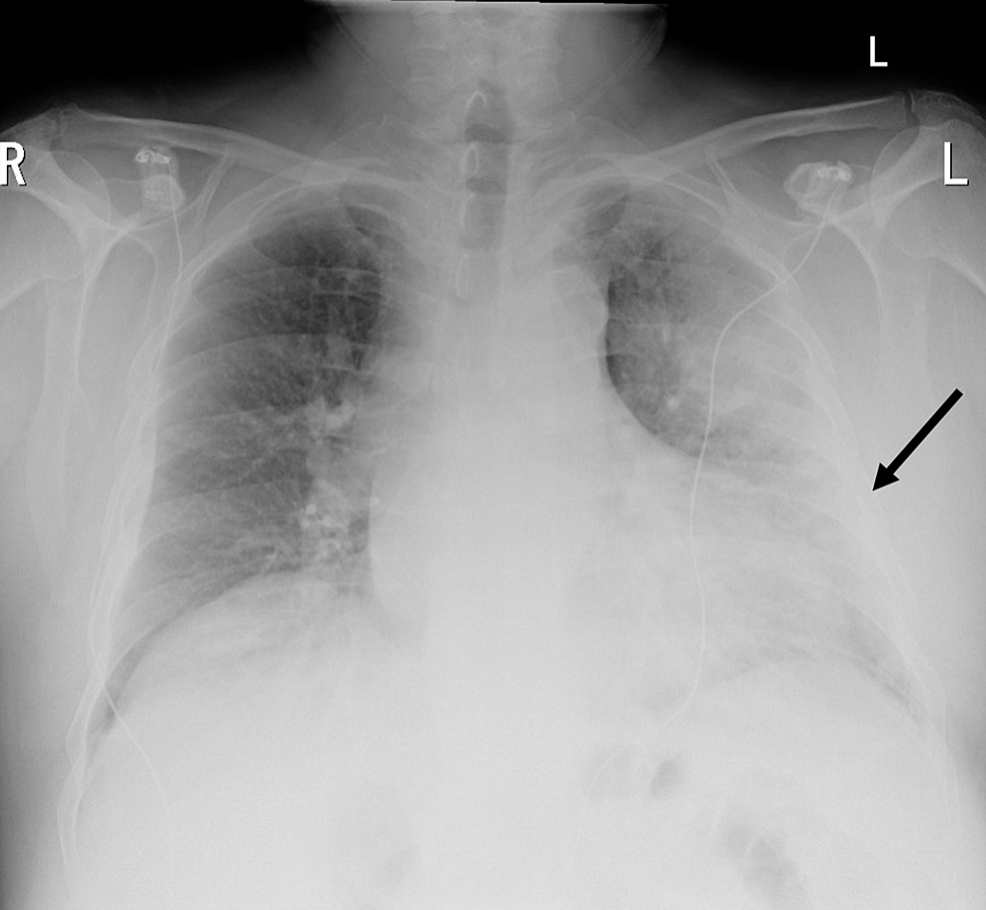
Chest X-ray Consolidation of left lung (black arrow)
